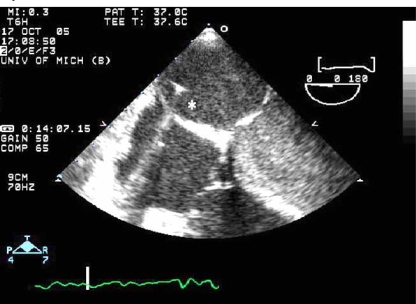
Atrial septostomy. An image of an atrial septal puncture is show above using transesophageal echocardiography during the procedure. Using a specialized catheter and fluoroscopy, an atrial septal puncture is made in the region of the fossa ovalis and balloon dilation is performed. The catheter (^*^asterix) can be seen traversing the septum from the right atrium into the left atrium.
